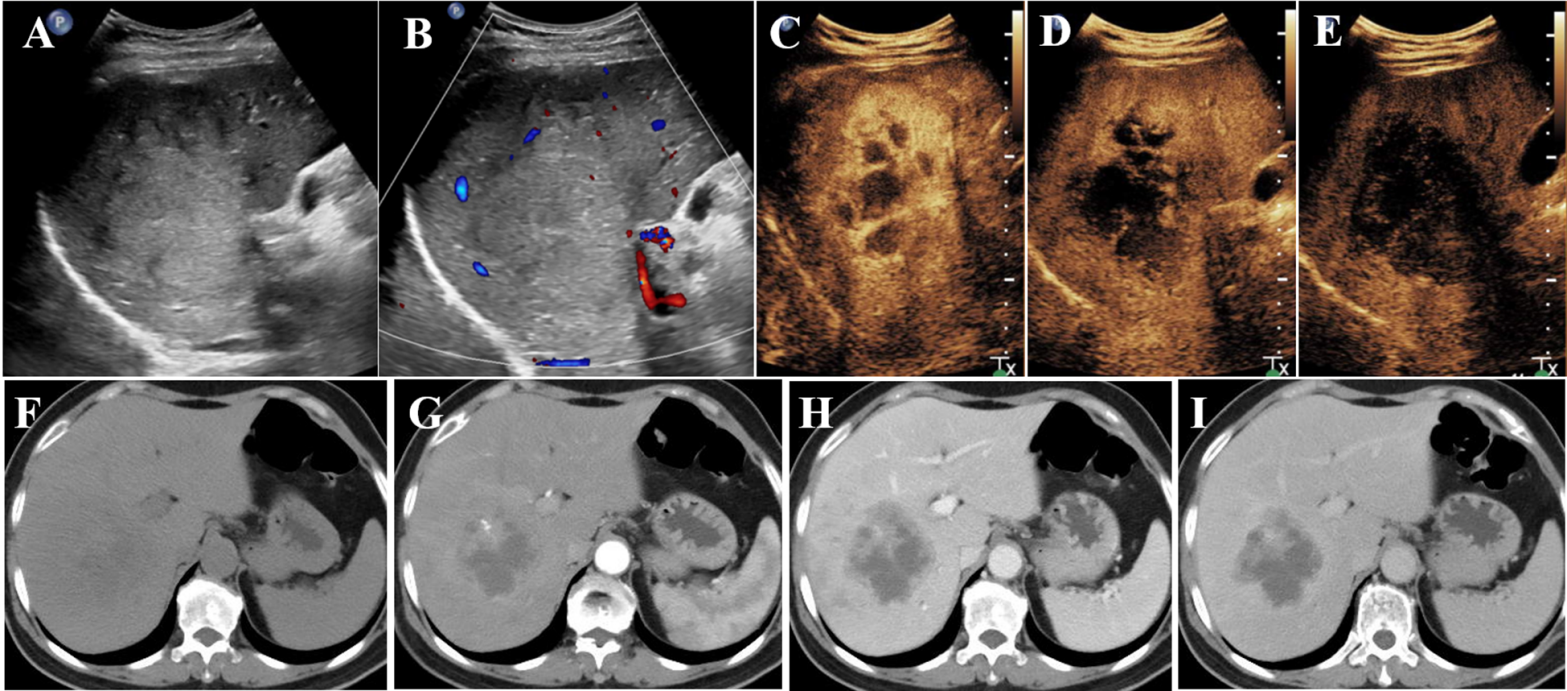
Conventional US revealed a mass (A, B) in the right lobe of the liver; CEUS showed irregular hyperenhancement with non-enhancement areas in the arterial phase (C), then washed out early in the portal venous phase (D), and finally marked washed out in the late phase (E). CT revealed a heterogeneous low-density mass in the right lobe of the liver (F); CECT showed mild-to- moderate heterogeneous enhancement in the arterial phase (G) and portal venous phase (H) in the mass. The enhancement degree was more obvious in the delayed stage (I). US, ultrasonography; CEUS, contrast-enhanced ultrasonography; CECT, contrast-enhanced computed tomography.
